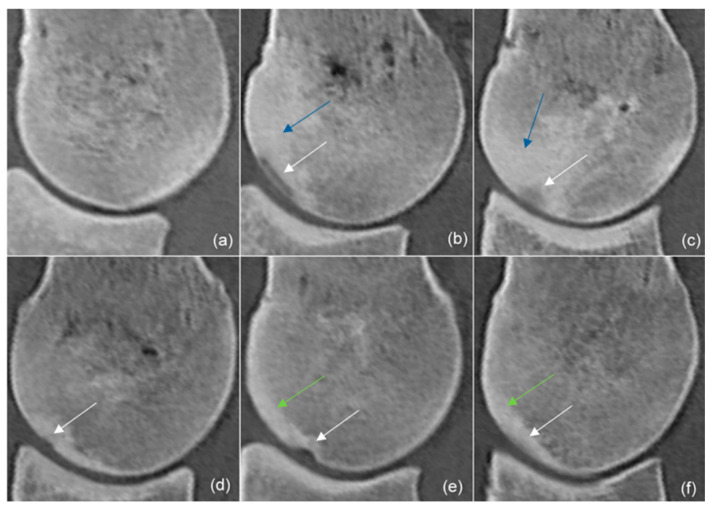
Sagittal computed tomographic reconstructions of the sagittal ridge of the third metacarpal bone (dorsal is to the left): a normal dorsal contour (a) and different shapes of hypoattenuating lesions (white arrows) in the subchondral bone of the sagittal ridge: proximodistally elongated lesions: the proximodistal extent of the lesion exceeds the dorsopalmar extent (b), lesions extending towards trabecular bone: the dorsopalmar extent of the lesion exceeds the proximodistal extent (c), uneven depressions: a depression in the subchondral bone surface with uneven margins (d), indentations: a depression in the subchondral bone surface with even margins (e), subtle lesions: minor hypoattenuation that did not fit the description of any of the categories above (f). Note the hyperattenuating area (blue arrow) surrounding the hypoattenuating lesion (b), the cone-shaped hyperattenuation (blue arrow) in the trabecular bone extending from around the hypoattenuating lesion, occupying more than half of the dorsopalmar extent of the bone (c), and the thickening of the subchondral bone plate (green arrow) (e,f).
